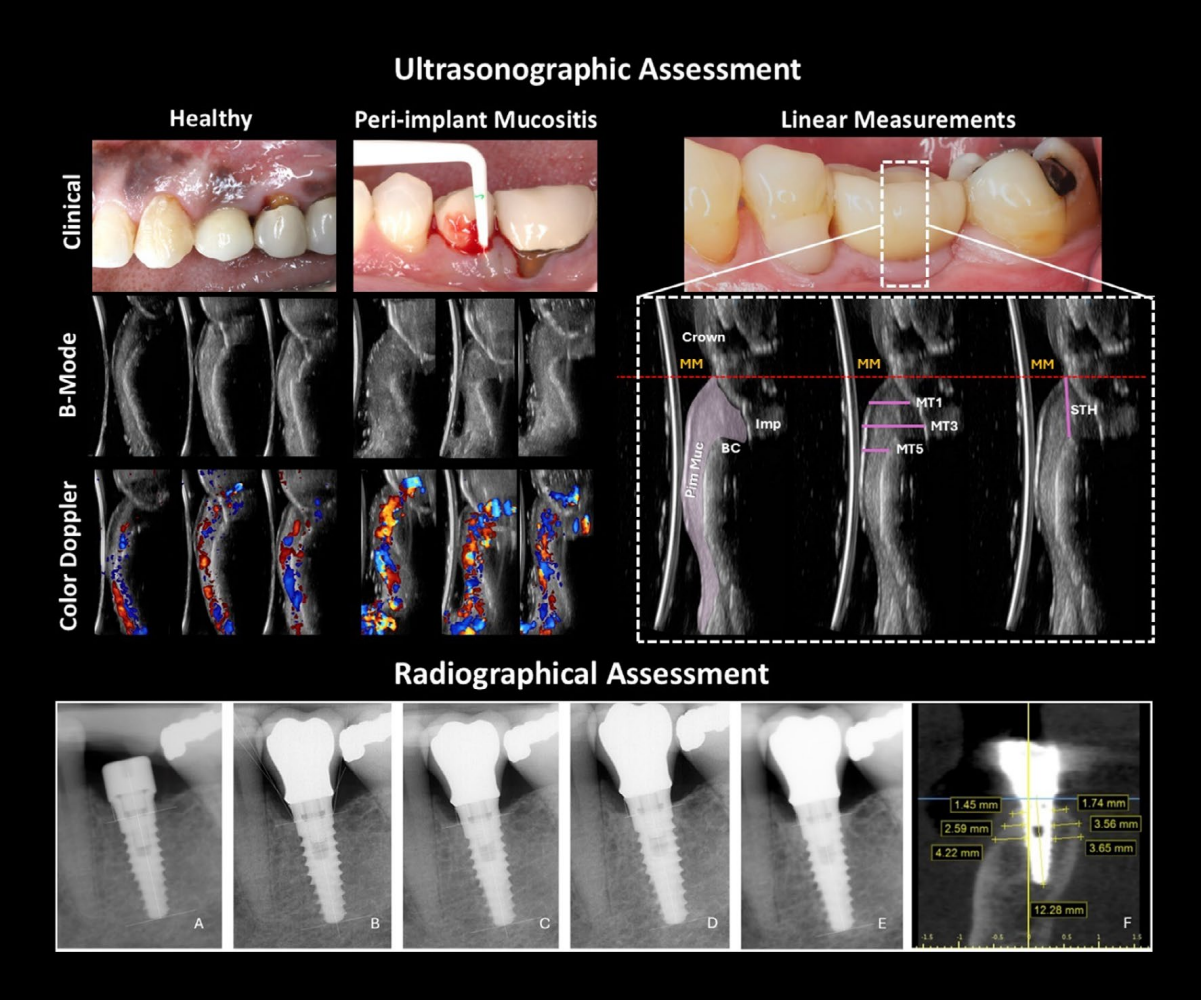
(top left): Clinical, B‐mode and C‐mode ultrasonographic images of a healthy and diseased implant. (top right): Ultrasonographic measurements. The red‐dashed line demarcates the mucosal margin (MM) around the implant. Peri‐implant mucosa (Pim Muc) is outlined as the pink area. Mucosal thickness (MT) was measured at 1 (MT1), 3 (MT3) and 5 (MT5) mm from the MM. Supracrestal tissue height (STH) was defined as the distance between MM and peri‐implant bone crest (BC). (Bottom): Standardised radiographic measurements. Peri‐apical x‐rays from baseline (A), crown delivery (B), 1 year (C), 3 years (D) and 5 years (E). The yellow lines show marginal bone level as well as emergence angle measurements. Buccal and labial bone thickness assessment from CBCT images (F). Radiographic assessments included standardised periapical radiographs with stent guidance and CBCT scans. Marginal bone‐level (MBL) changes were calculated from mesial and distal measurements (ImageJ), with high intra‐examiner reliability (ICC = 0.92). Three‐dimensional CBCT analysis quantified buccal and palatal bone thickness at 2, 4 and 6 mm below the implant platform.
